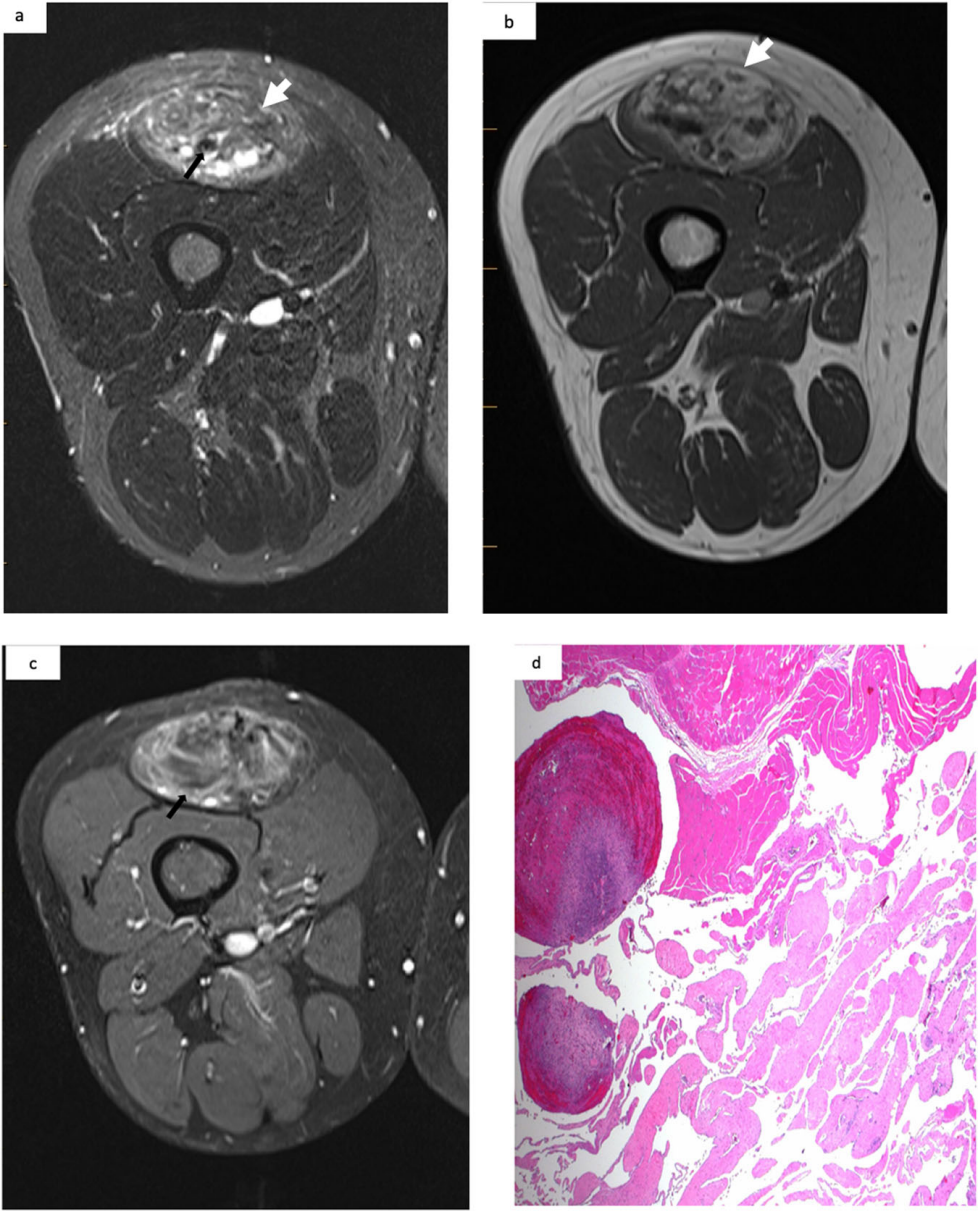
MRI of a 72-year-old woman with a painful mass on her right thigh: a Heterogeneous hyperintensity appearance of the intramuscular mass on T2WI with fat suppression (white arrow) with phleboliths (black arrow). b Heterogenous hyperintensity appearance of the mass on T1WI (white arrow). c Moderate enhancement (black arrow) on delayed contrast-enhanced T1WI with fat suppression. d A thrombosed venous malformation indicated by pathological analysis
